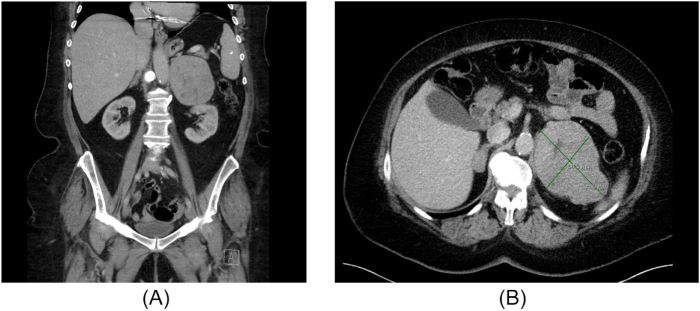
Figure A and B show axial and coronal CT scans, respectively. A slightly heterogenous mass of 8.4 cm by 11.2 cm is seen in the left adrenal gland.
